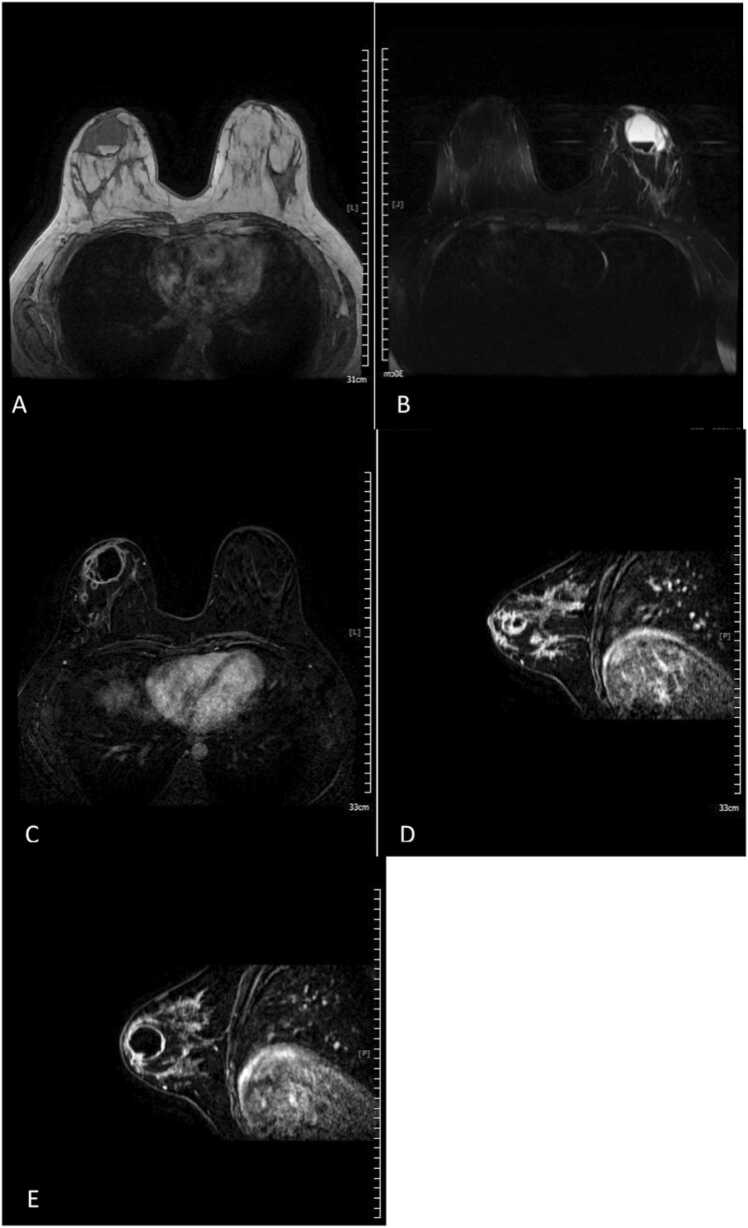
Axial T1-weighted (A), Axial T2-weighted (B), and Axial and sagittal post contrast T1 (C–E). Images show a thick rim enhancing blood fluid cystic mass in right breast retro areole region along with multiple smaller cysts in surrounding parenchyma.
